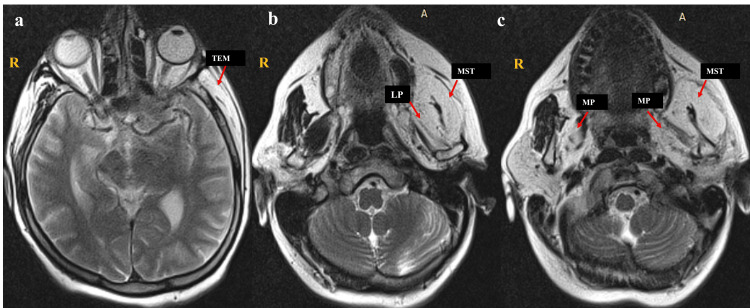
Axial T2-Weighted MRI Demonstrating Chronic Denervation Changes in the Muscles of Mastication Axial T2-weighted non-fat-saturated MR images obtained at the level of the temporalis (a), lateral pterygoid (b), and medial pterygoid (c) muscles demonstrate persistent hyperintense fatty signal alteration with marked volume loss involving the left temporalis (TEM), masseter (MST), lateral pterygoid (LP), and medial pterygoid (MP) muscles, consistent with chronic denervation changes. Fatty infiltration and mild volume loss are again noted within the right medial pterygoid muscle. R = right side.
